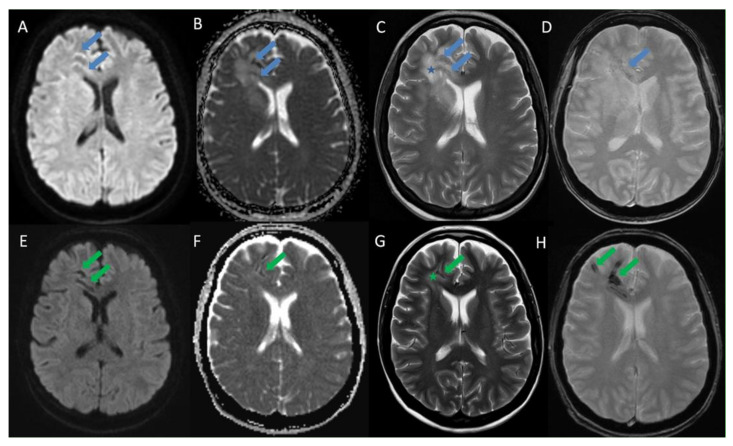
Initial MRI brain at admission (upper row) vs MRI brain 4 weeks after treatment (lower row) at the level of lateral ventricles: Axial DWI (A and E), Axial ADC (B and F), Axial T2 (C and G) and Axial T2* (D and H): The initial scan in the upper row shows no true restricted diffusion but prominent ADC hypointensities corresponding to the lesions which are thought to be secondary to subtle punctate foci of susceptibility artifacts/inflammatory free radicles (blue arrows). Surrounding vasogenic edema in the brain parenchyma is noted (blue star). The follow-up MRI 4 weeks after treatment shows an increase in the susceptibility artifacts blooming along the lesions on the T2* sequence with corresponding reduction of T2 signals and ADC values (green arrows). The surrounding vasogenic edema is also decreased (green star).
